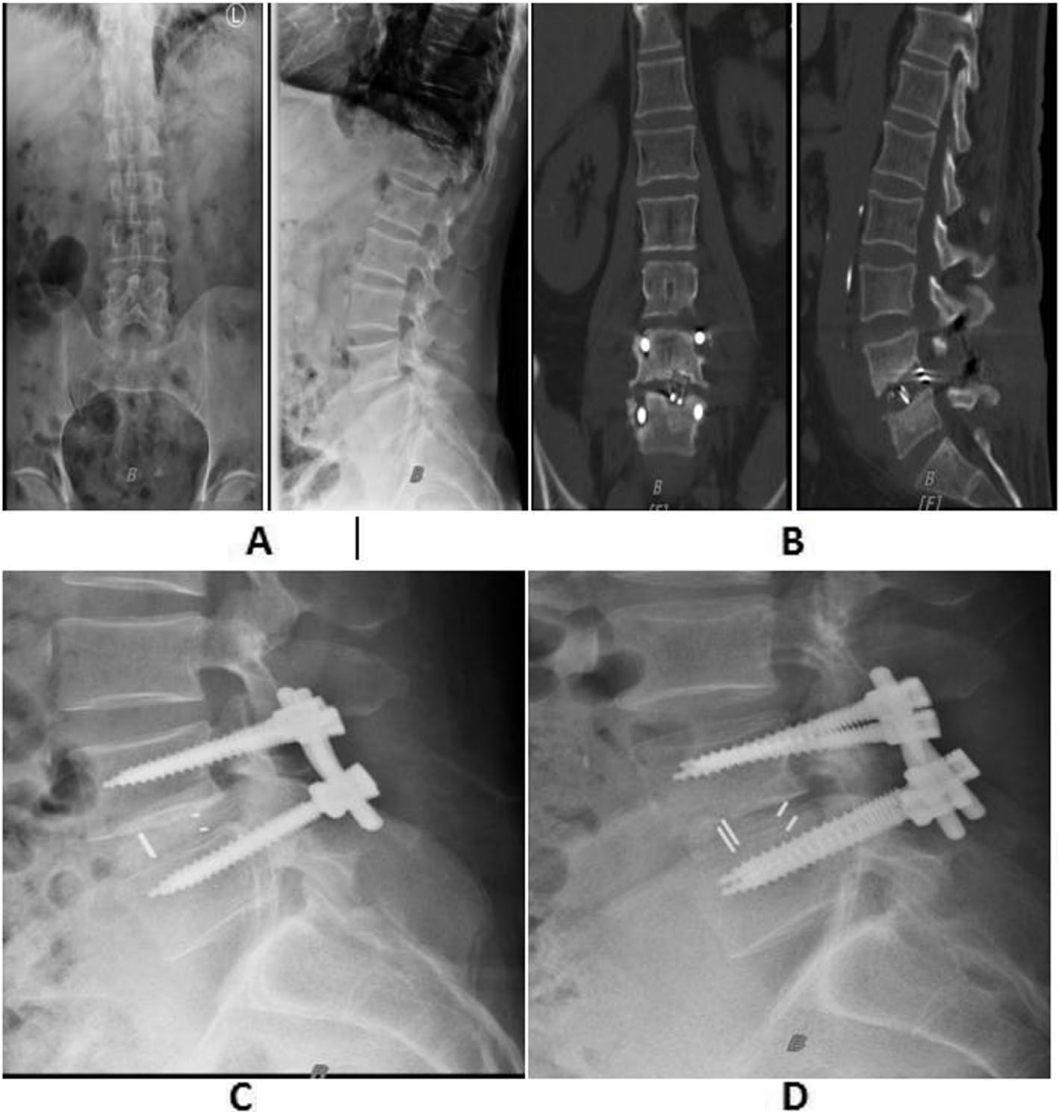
A 66-year-old osteoporotic woman with lumbar spondylolisthesis (forearm T-score = − 2.8) underwent PLIF. A Preoperative anterior–posterior and lateral lumbar X-rays. B Measurement of cage subsidence distance on CT mid-sagittal and coronal images at 15 months postoperatively. C Lateral lumbar X-rays at 1 week postoperatively. D Cage subsidence on X-ray at 1 year postoperatively
